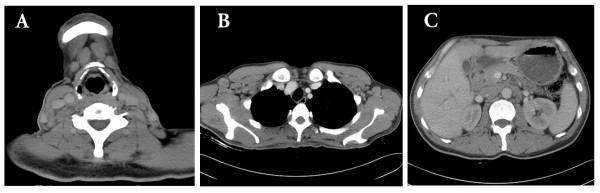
Computed tomography scanning of the neck, chest and abdomen (A). Enlargement of numerous lymph nodes in the neck. (B). Numerous enlarged lymph nodes in axillary space. (C) Enlargement and confluence of lymph nodes in the peritoneal cavity.
